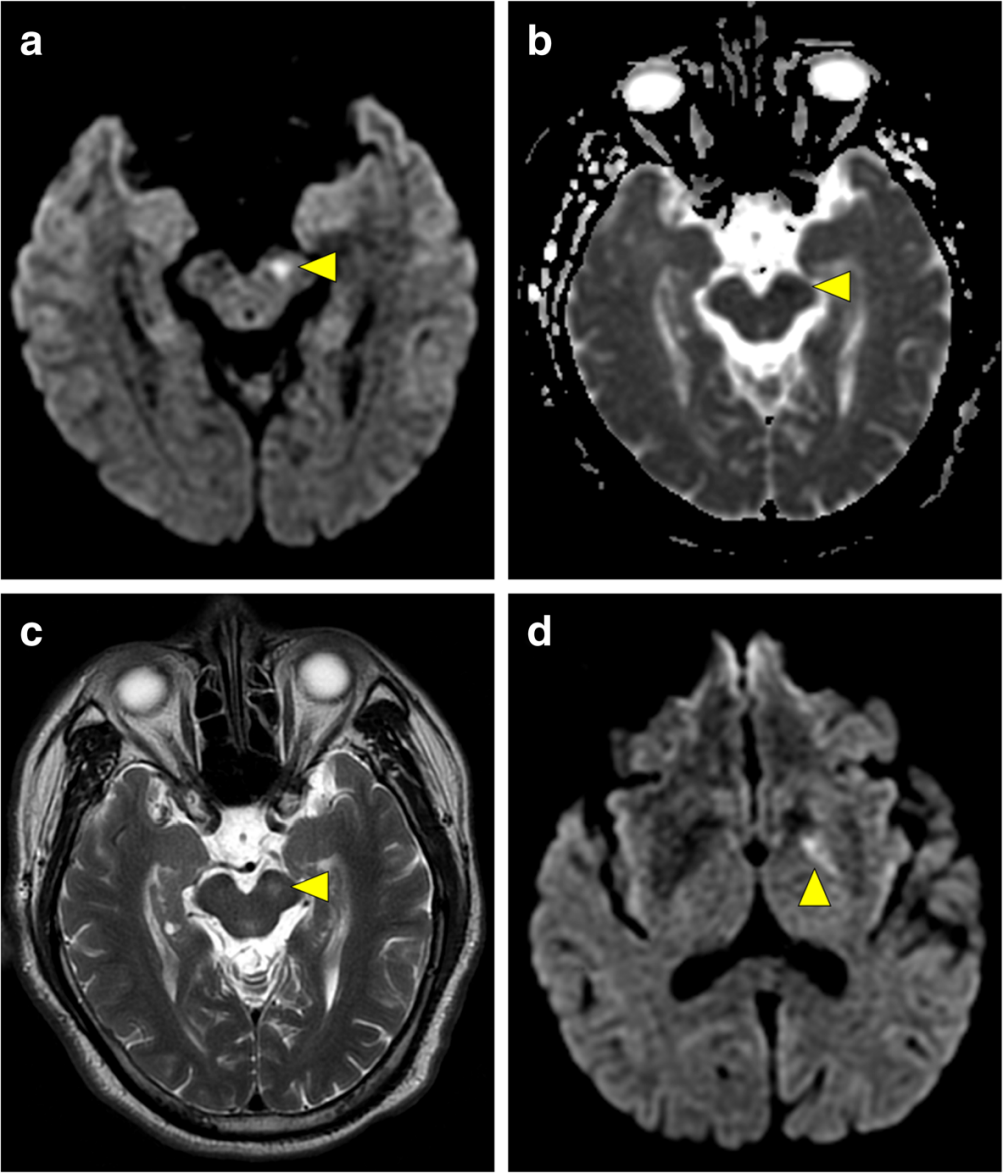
MR scans of the brain at the level of the midbrain and thalamus revealed hyperintense changes on DWI and T2WI in the left SN and globus pallidus (a, c and d, arrowheads) with corresponding low signal intensity on the apparent diffusion coefficient map (b, arrowhead). The findings indicated acute pallidonigral degeneration secondary to striatonigral pathway disruption
